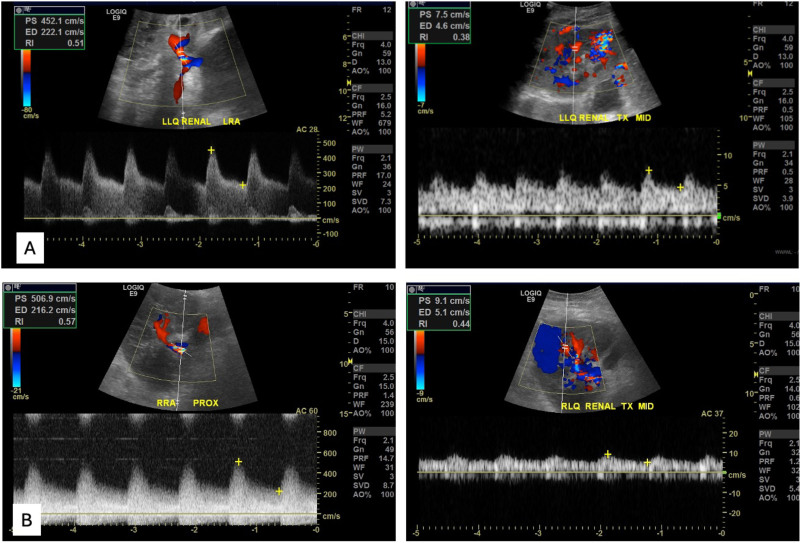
Doppler ultrasound evaluation of transplant renal arteries. A,Case 1 Doppler ultrasound: markedly elevated mid-renal artery velocity and reduced intraparenchymal diastolic flow, consistent with hemodynamically significant stenosis/compression. B, Case 2 Doppler ultrasound: markedly elevated mid-renal artery velocity and reduced intraparenchymal diastolic flow, consistent with hemodynamically significant stenosis/compression.
